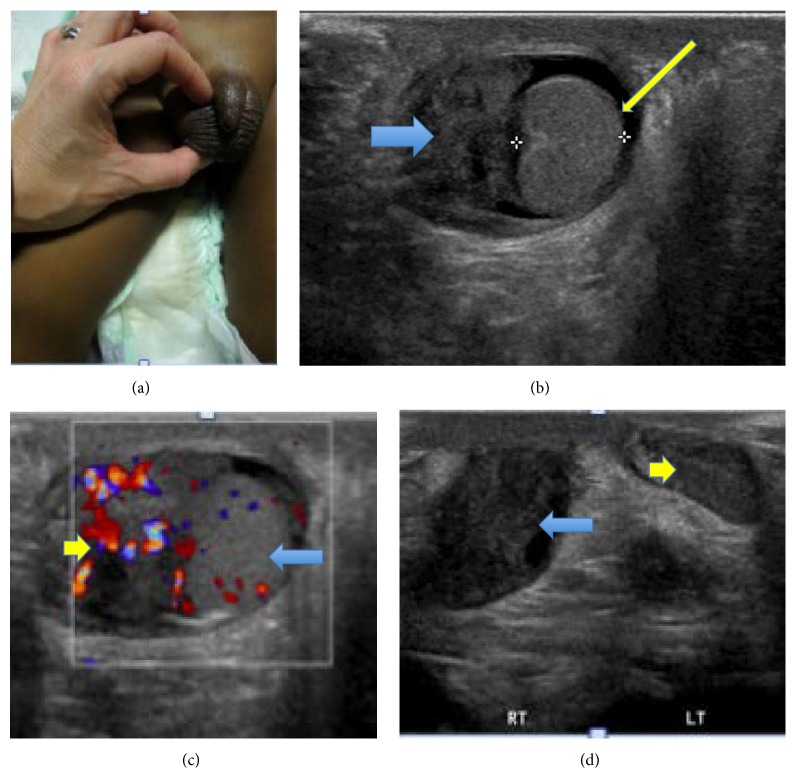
Physical exam and imaging of right testis at diagnosis. (a) Enlarged right testis. (b) Baseline ultrasound of the scrotum demonstrated enlarged right testis (thin yellow arrow) and epididymis (thick blue arrow). (c) Testicular Doppler showing hyperemic right testis (long blue arrow) and epididymis (short yellow arrow). (d) Right side testicle (long blue arrow) was estimated to be approximately twice the volume of the left (short yellow arrow).
